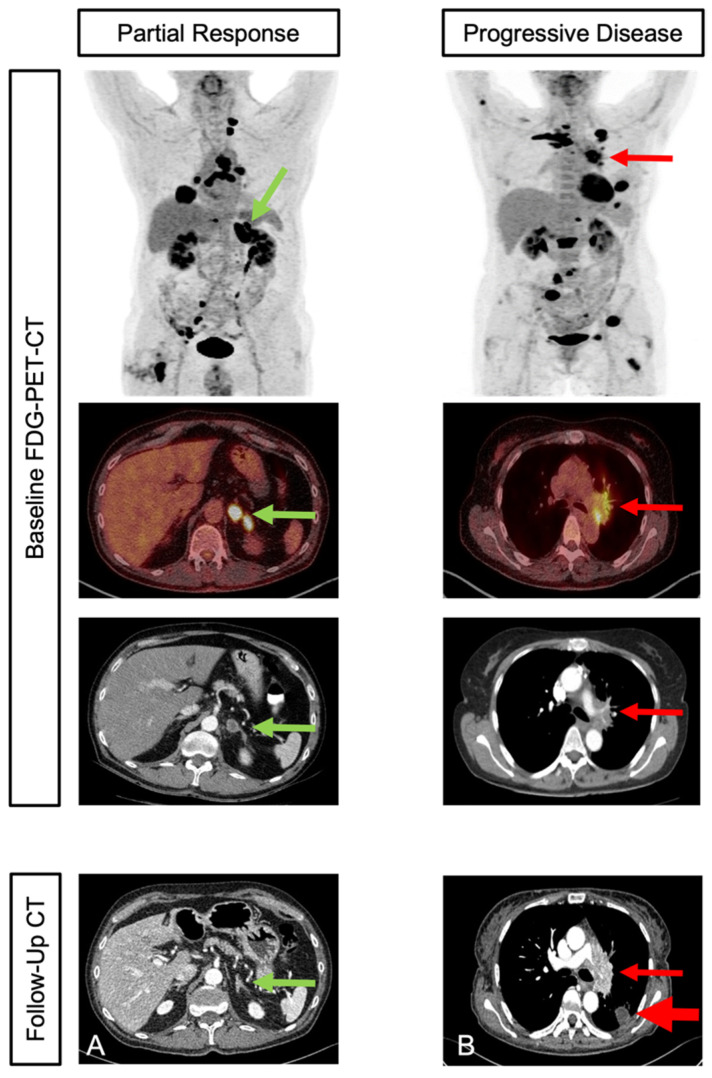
Initial staging and second follow-up in two patients with different response: Patient with adrenal (marked with green arrow), bone, and lymph node metastases in initial staging PET-CT. Partial response was shown in contrast-enhanced CT in second follow up (A). Patient with multiple intra-pulmonal (marked with red arrow) and bone metastases in initial staging PET-CT. Progressive disease was shown in contrast-enhanced CT in second follow up (B).
